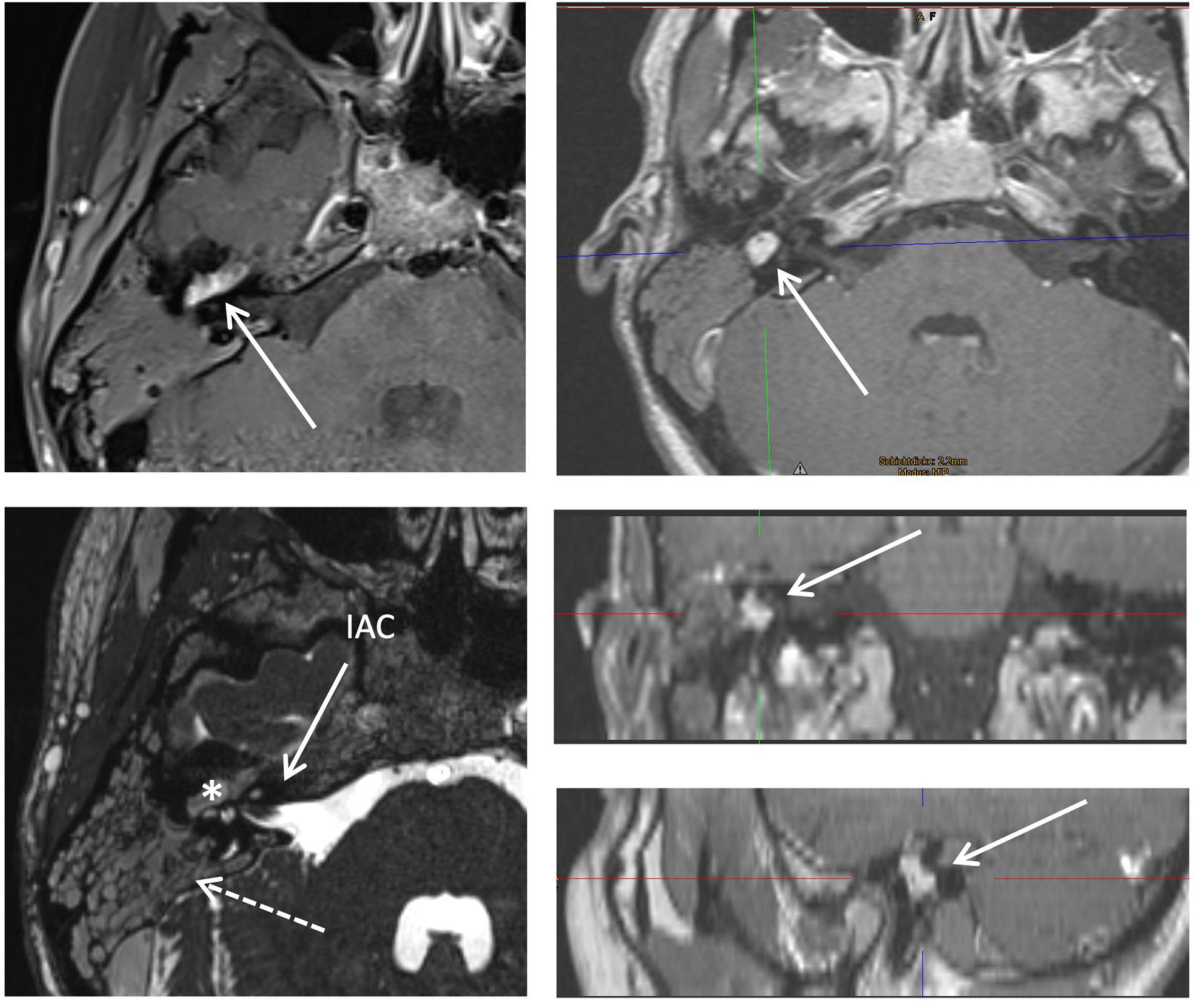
Preoperative cMRI. Top left: Axial T1-weighted sequence showing thickening of the mastoid and contrast enhancement of the epitympanic segment of the facial nerve (arrow). Bottom left: Axial high-resolution T2-weighted 3D sequence demonstrating complete opacification of the right mastoid (dashed arrow) and a ventilation disorder caused by a sharply circumscribed hyperintense mass (*) in the mastoid antrum. IAC, internal auditory canal. Top, middle and bottom right: Axial, coronal and sagittal T1-weighted sequence showing schwannoma of the facial nerve (arrows).
